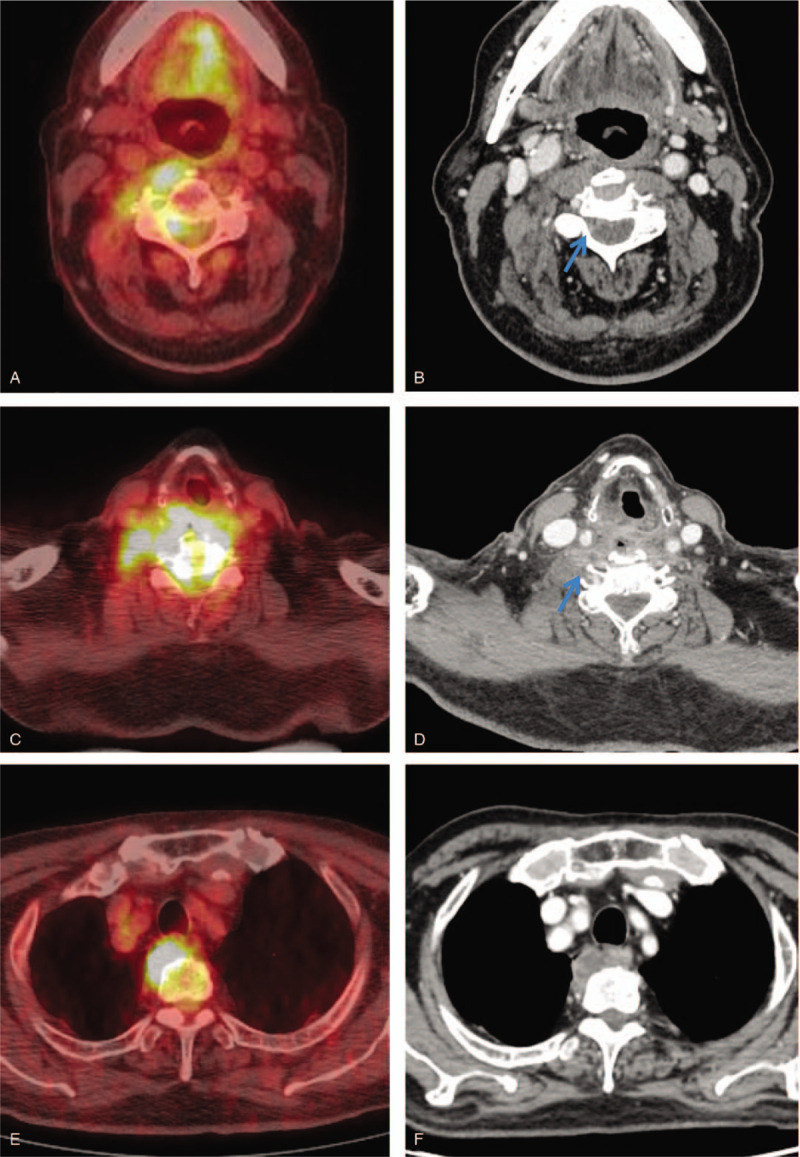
Axial images of ^18^F-fluorodeoxyglucose positron emission tomography/computed tomography (FDG PET/CT) (A, C, and E) and contrast-enhanced CT (B, D, and F) reveal hypermetabolic lesions with mild contrast enhancement along the danger and right paravertebral spaces corresponding to deep neck infections (A, B, C, and D). PET/CT reveals related osteomyelitis of the vertebral body of C4 with intense FDG uptake without significant bone changes on CT (C and D). Hypermetabolic lesions along the nerve root and spinal canal are also seen with mild contrast enhancement on CT (B and D, blue arrow). Continuous necrotizing mediastinitis with intense FDG uptake is observed along the prevertebral space of the upper mediastinum. On axial CT, central necrotic material is observed as a low-attenuation lesion (E and F).
